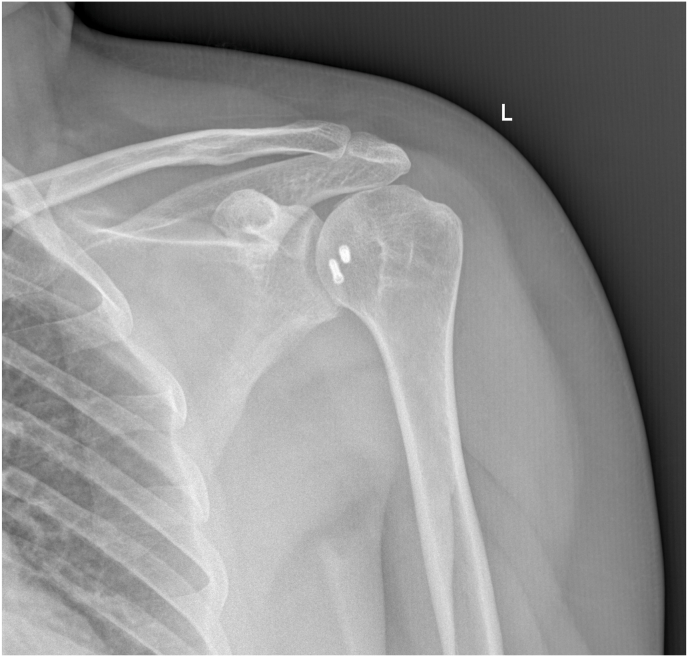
Anteroposterior X-ray of the left shoulder in a 28-year-old woman after the Modified McLaughlin procedure, fixation with 2 cannulated screws.
